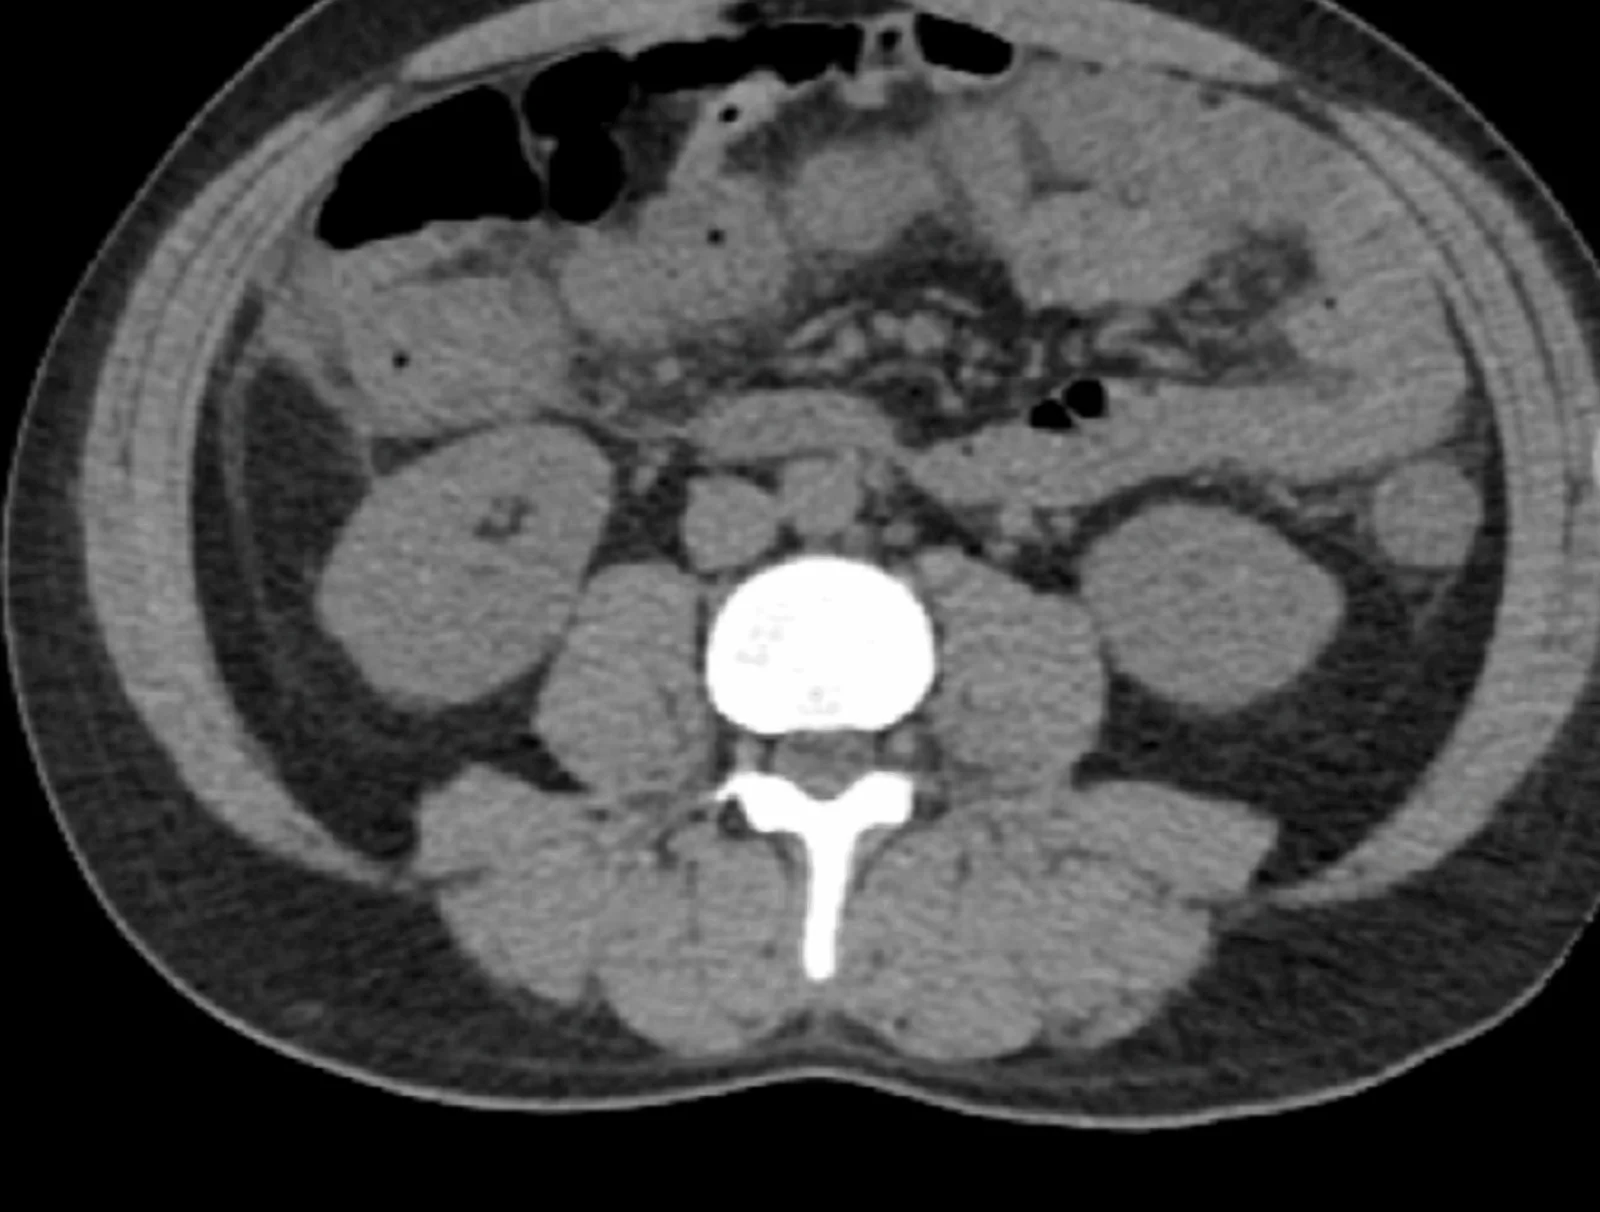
CT axial displaying colonic inflammation and fat stranding.
